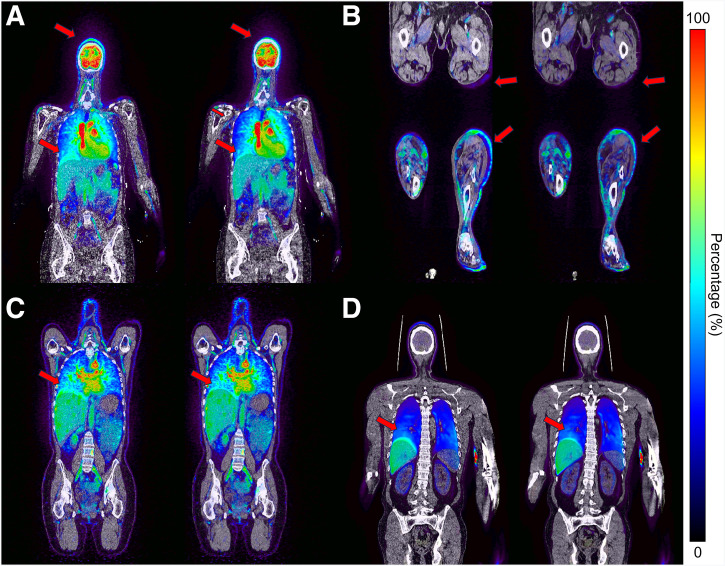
(A) ^15^O-H_2_O torso, (B) ^15^O-H_2_O legs, (C) ^89^Zr-trastuzumab torso, and (D) ^18^F-MC225 torso fused NAC-PET images with original AC-CT (left) and coregistered CT (right). Reduced mismatch between PET and CT indicated by red arrows.
